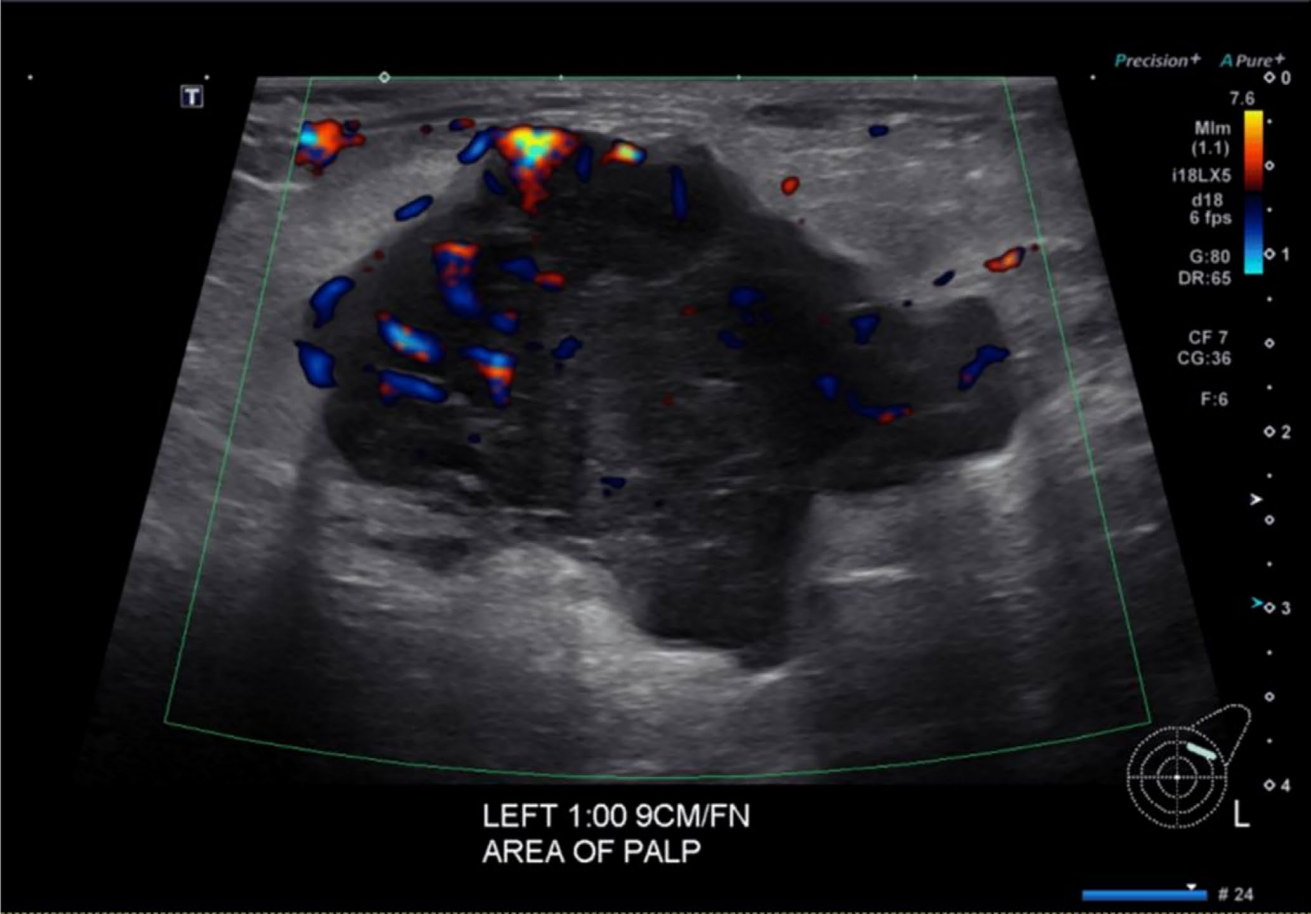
Left breast mass and axillary lymphadenopathy on ultrasound. Ultrasound of the left breast revealed an irregular mass measuring 4.2 × 3.7 × 2.9 cm at the 1 o'clock position, located 9 cm from the nipple, corresponding to the major lesion seen on mammography. Additionally, multiple enlarged lymph nodes were present at the left axilla, demonstrating loss of the normal fatty hilum. BI‐RADS 5.
